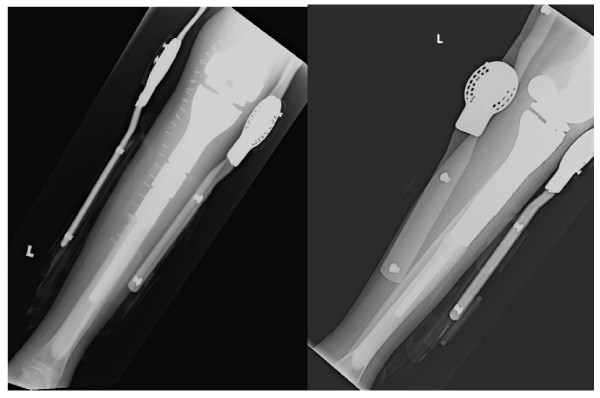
Postoperative radiographs showing the implant within the bone with an external knee brace.
